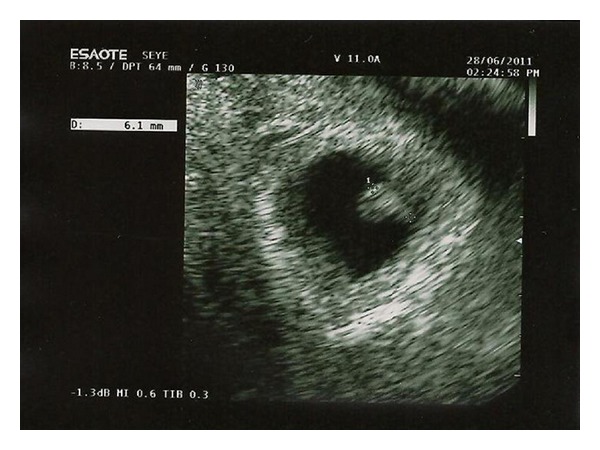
Transvaginal ultrasound of uterus showing a gestational sac of approximately 7 weeks.
